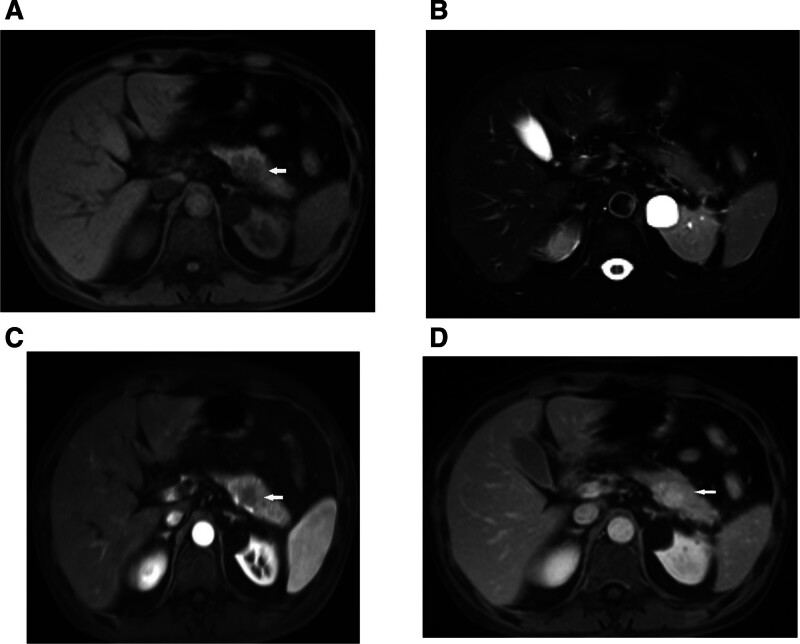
Magnetic resonance imaging (MRI) findings of the pancreatic tail mass. (A) T1-weighted image; (B) T2-weighted image; (C)arterial phase; (D) portal venous.
